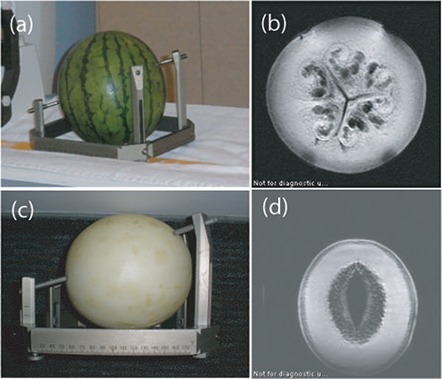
Photograph and associated MRI images of a watermelon (a) and (b), and a honeydew melon ((c) and (d)). Head‐frame screws should be kept in the shell region as indicated in the MRI images in order to maintain resistance in the MΩ range and thus be comparable to the human head.
